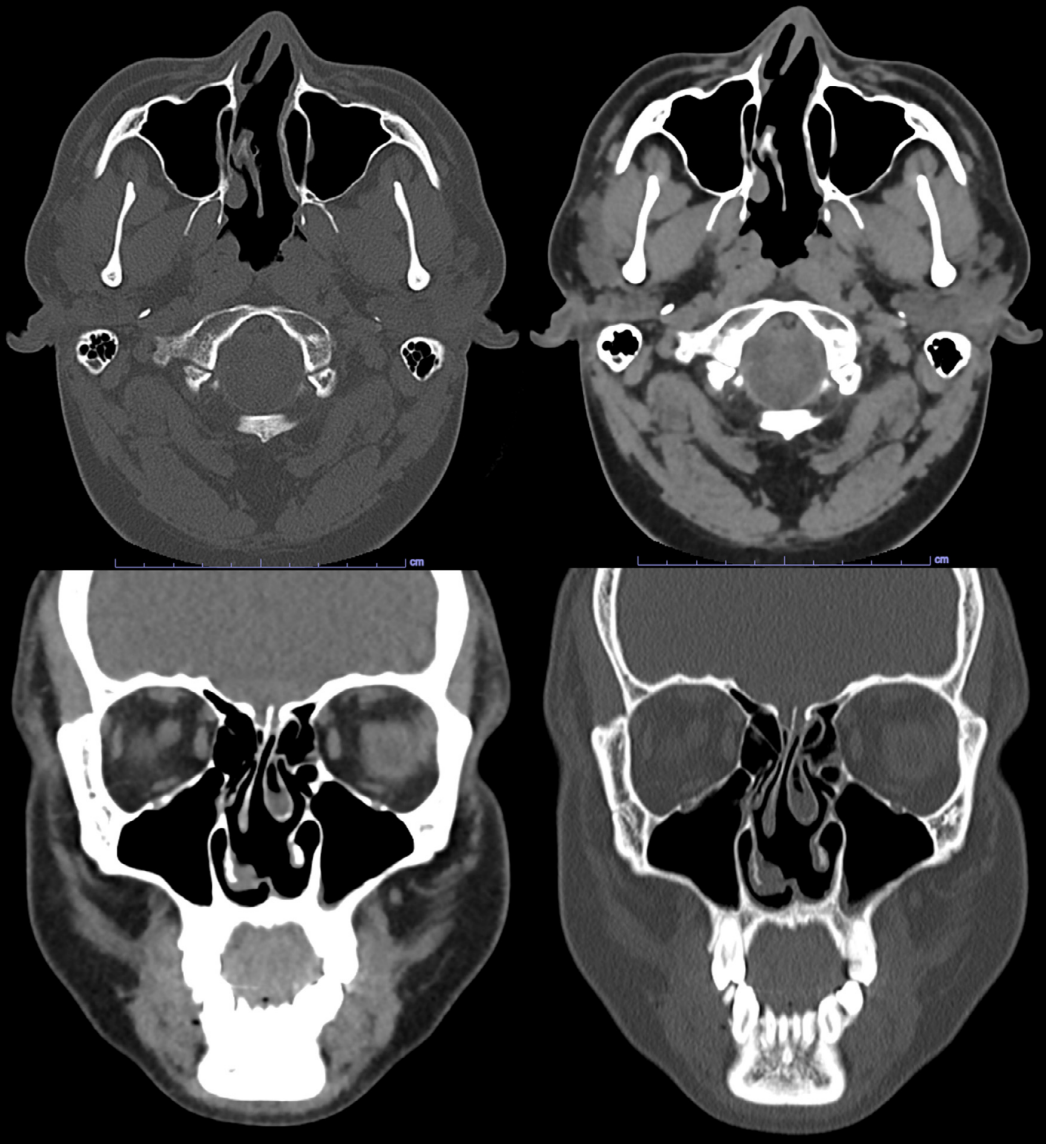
CT Sinus protocol demonstrates erosion of the anterior cartilaginous portion of the nasal septum
